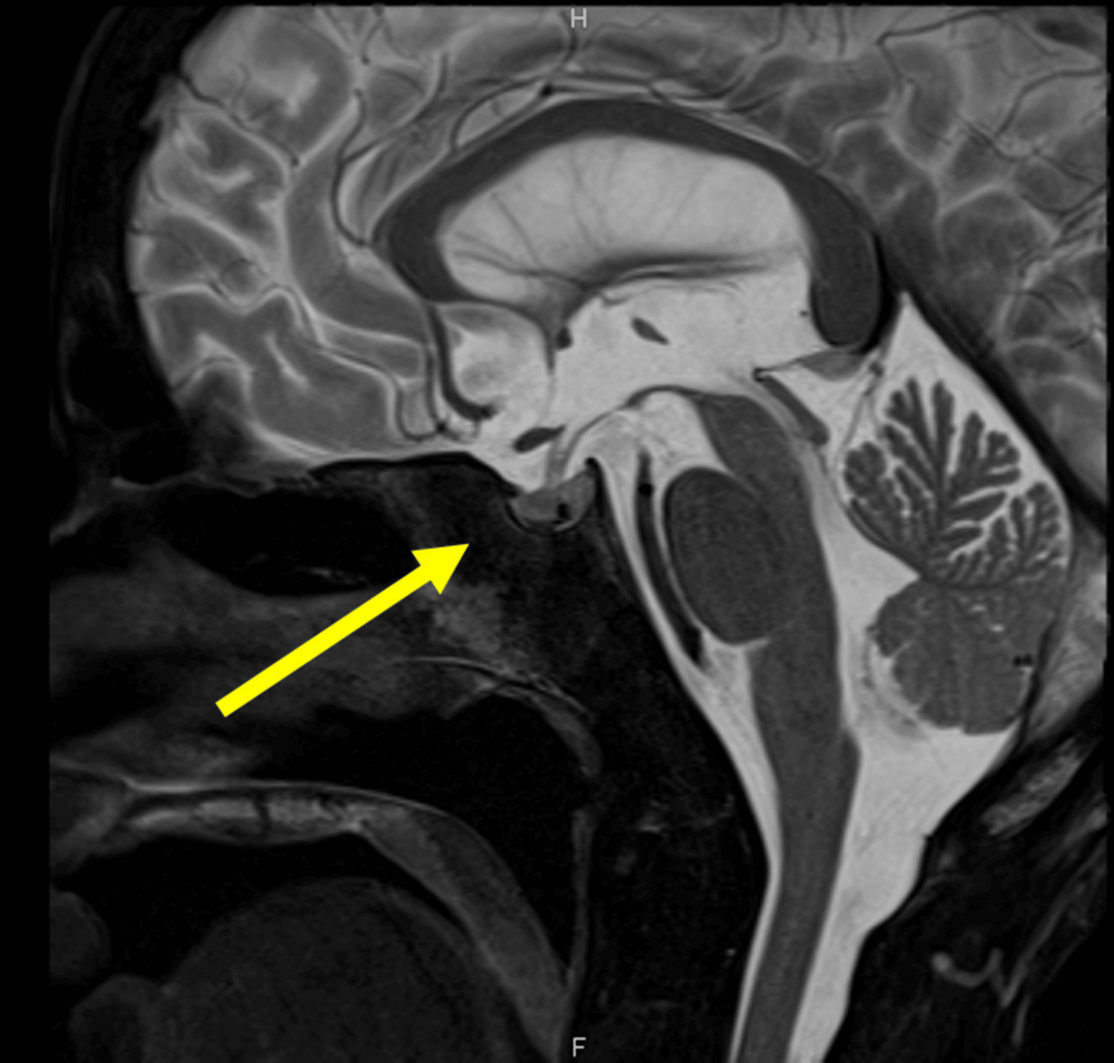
MRI of the head shows no significant findings in the pituitary gland.
